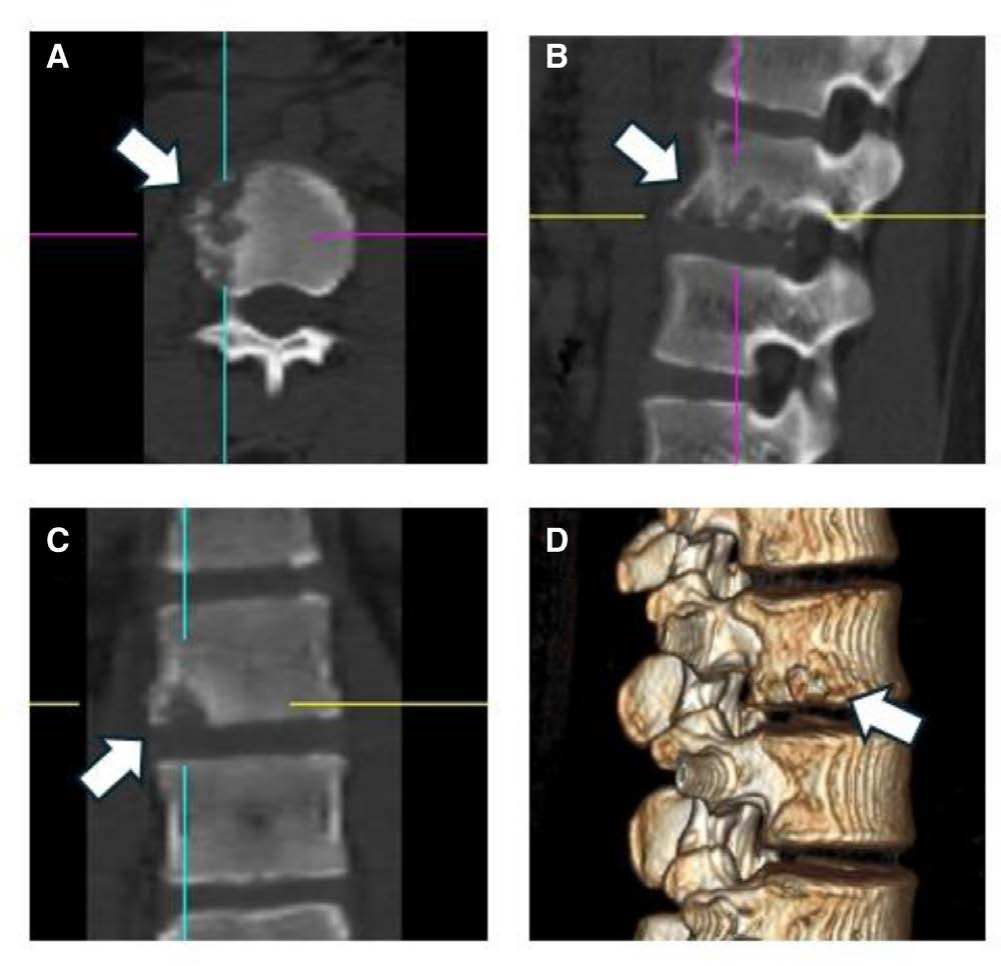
CT images obtained before admission. Horizontal (A), sagittal (B), and coronal (C) CT images and 3D reconstruction (D) show the osteolytic changes in the lesion bone. CT = computed tomography.
